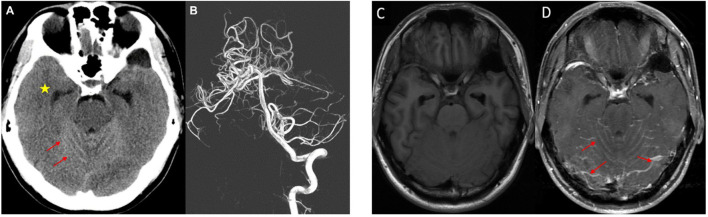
(A) Non-contrast head CT demonstrating diffuse hyperattenuation within the posterior fossa sulci (red arrows), mimicking subarachnoid hemorrhage. Note the dilated temporal horn of the lateral ventricle (yellow star), indicating early obstructive hydrocephalus. (B) Digital subtraction angiography showing no evidence of aneurysm or vascular malformation. (C) Pre-contrast T1-weighted MRI at the posterior fossa level demonstrating no abnormal leptomeningeal signal hyperintensity, a finding atypical for melanin-containing melanocytic lesions and representing the key diagnostic pitfall of this amelanotic variant. (D) Post-contrast T1-weighted MRI at the same level revealing diffuse leptomeningeal enhancement along the posterior fossa sulci and cerebellar folia (red arrows), consistent with extensive melanocytic infiltration.
